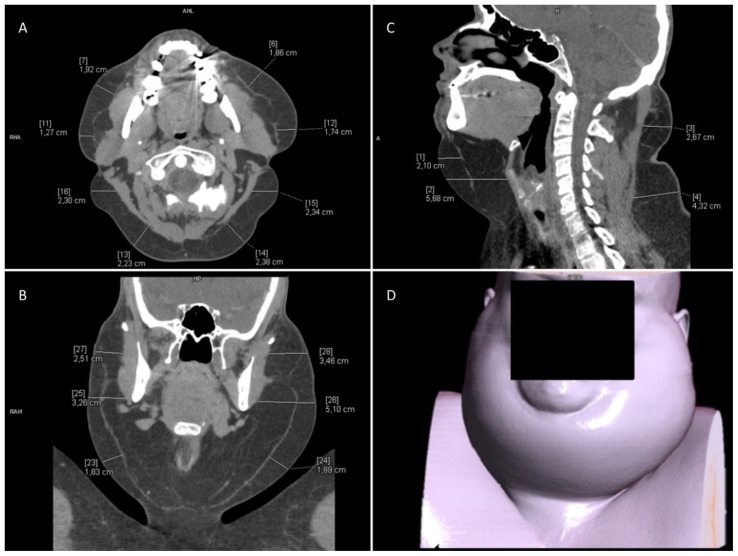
Computed tomography of the neck. (A) Axial reconstruction. (B) MPR reconstruction, frontal view. (C) MPR reconstruction, sagittal view. (D) VRT reconstruction.
